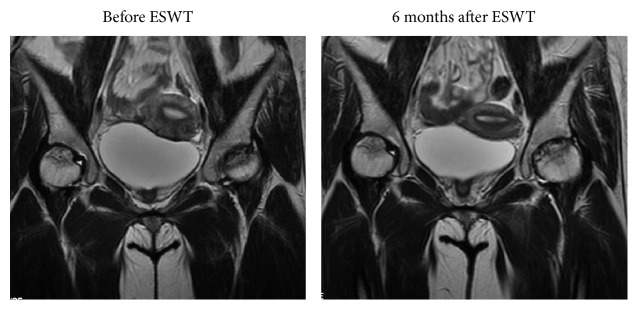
MR images of the bilateral hips in a 31-year-old female patient with glucocorticoid-induced osteonecrosis of the femoral head showed regression of the lesion 6 months after ESWT, and the hips were pain-free for daily activities.
